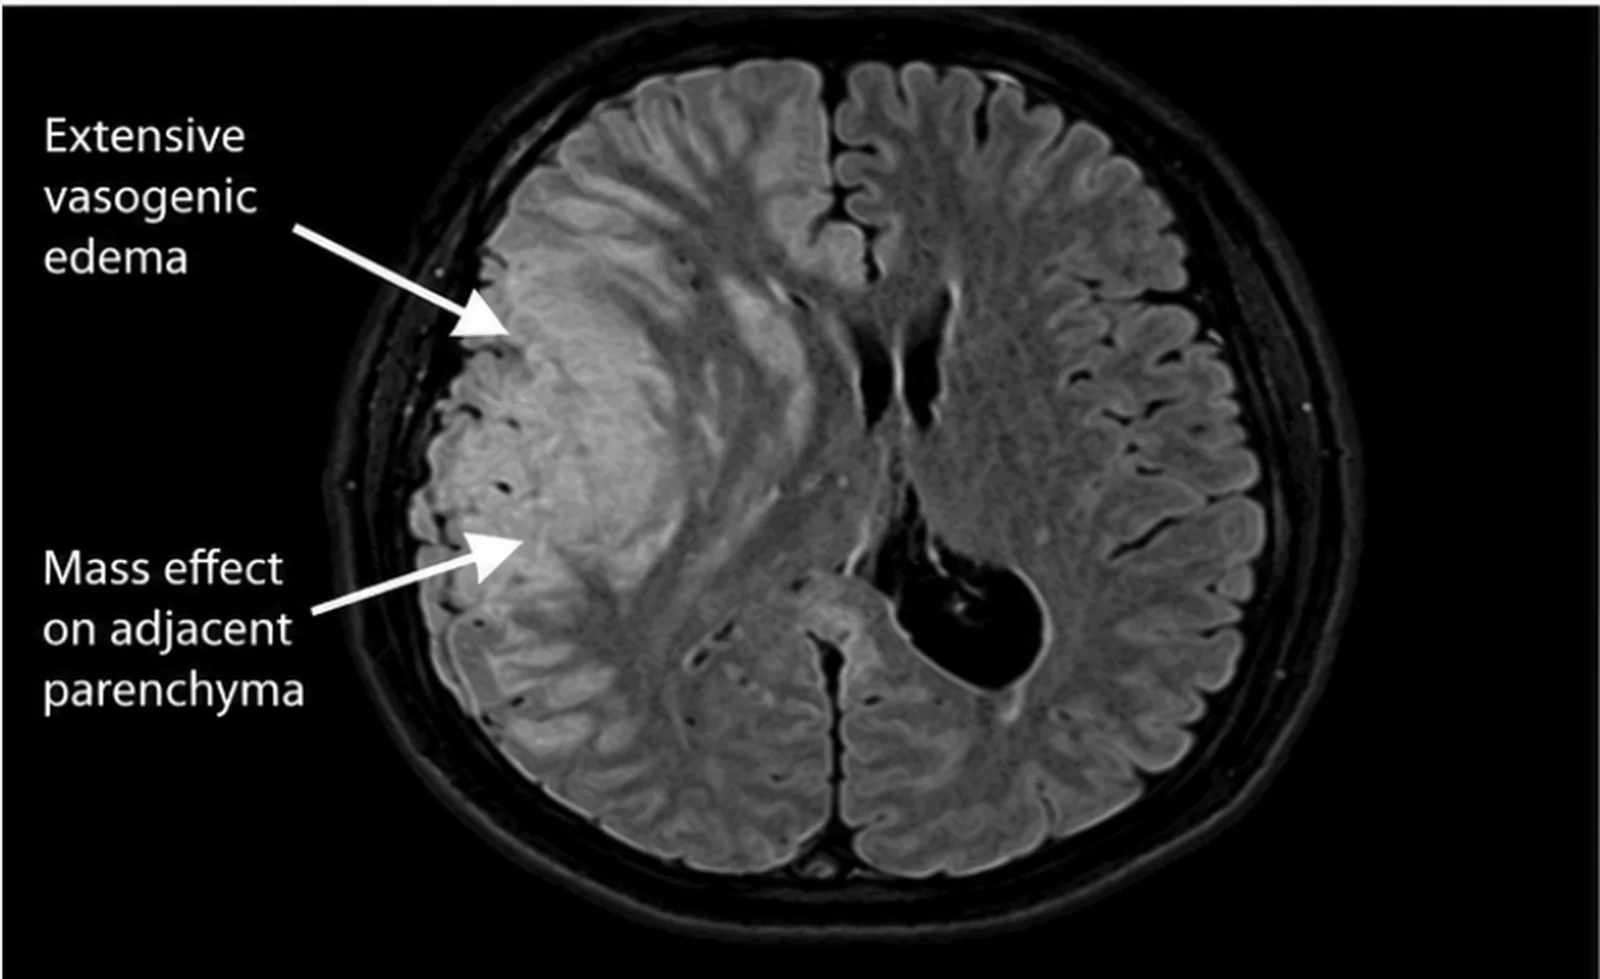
Preoperative FLAIR MRI. Axial fluid-attenuated inversion recovery (FLAIR) MRI demonstrating extensive surrounding vasogenic edema (white arrow) with significant mass effect on the adjacent brain parenchyma (arrowhead).
